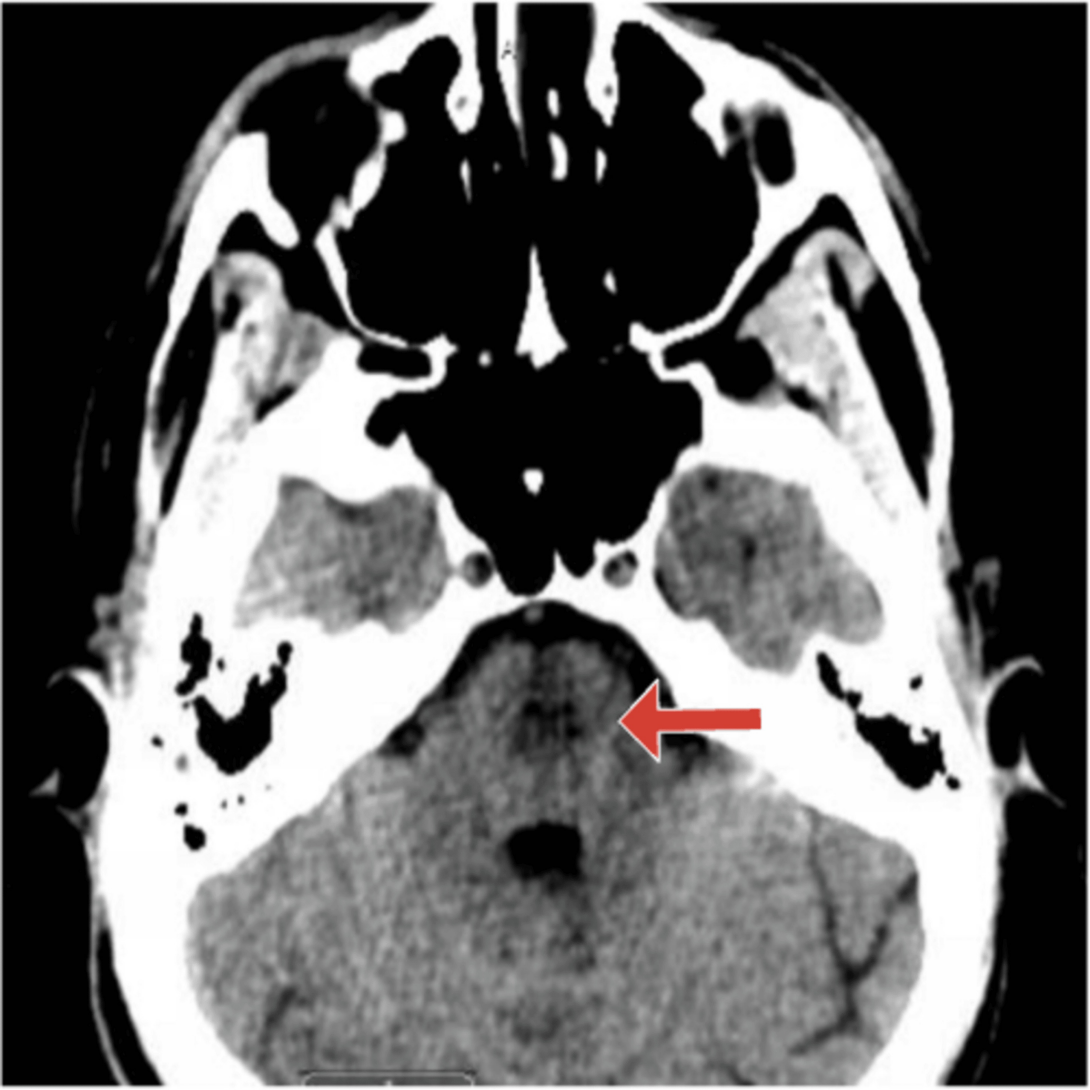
Head CT The image shows a hypodense area within the mid-pons (arrow), warranting further evaluation with MRI CT: computed tomography; MRI: magnetic resonance imaging
